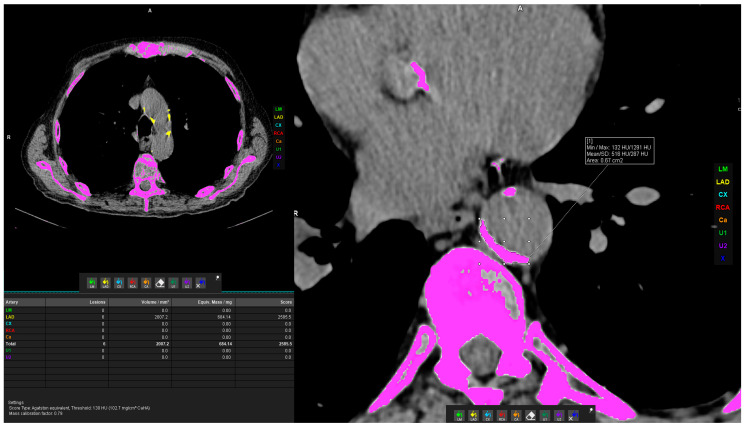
Axial chest CT scans of calcification measurement. (Left) Thoracic aorta calcification (TAC) measurement using postprocessing software VB60_HF08. (Right) Freehand ROI measurement of calcification volume.
